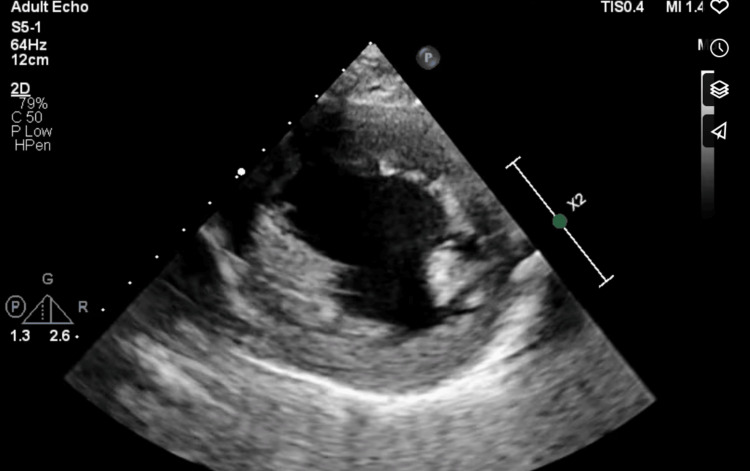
Parasternal short axis view revealing concentric left ventricular hypertrophy and trabeculation
